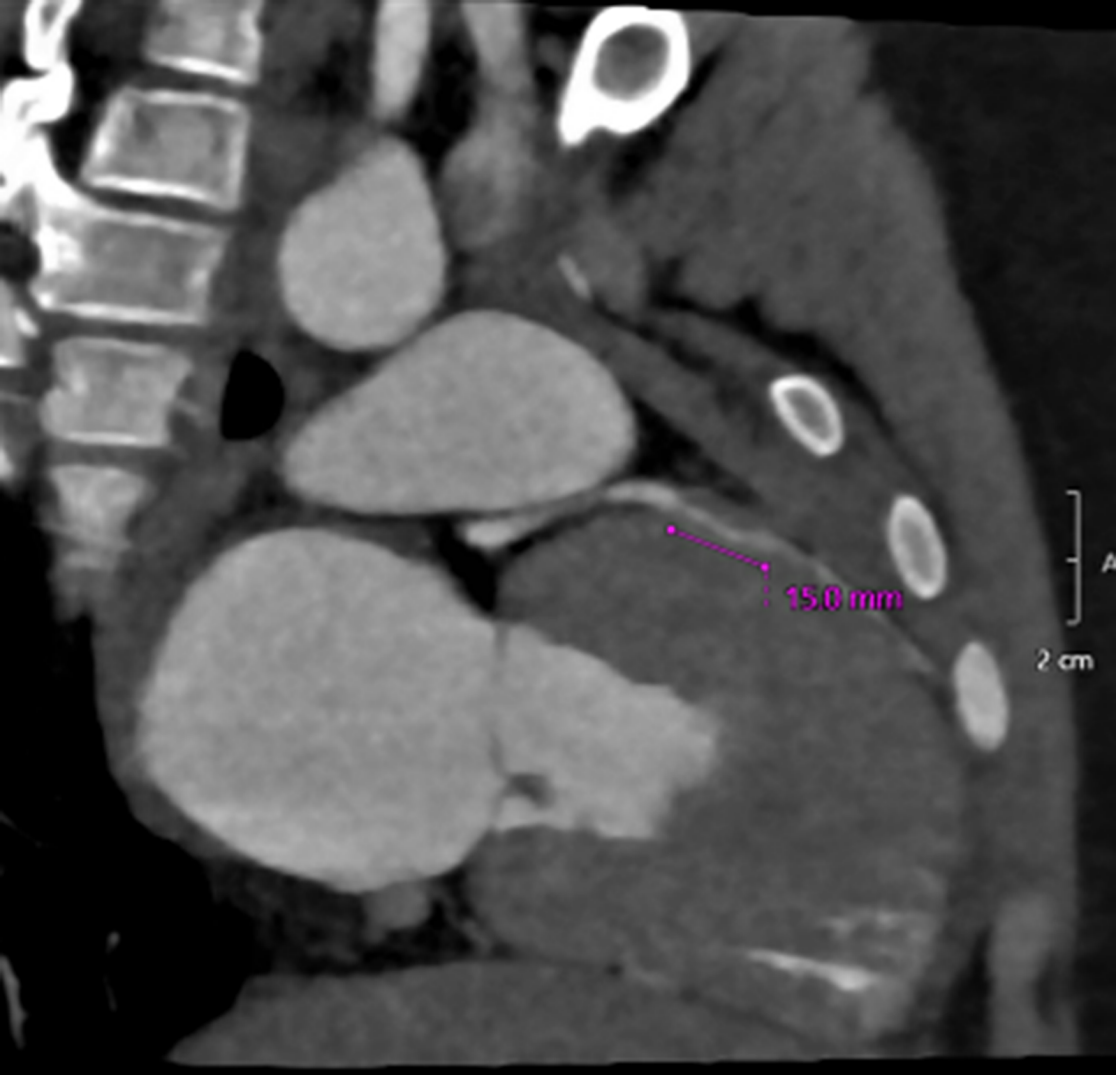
CTA image showing a left anterior descending coronary artery myocardial bridge.
